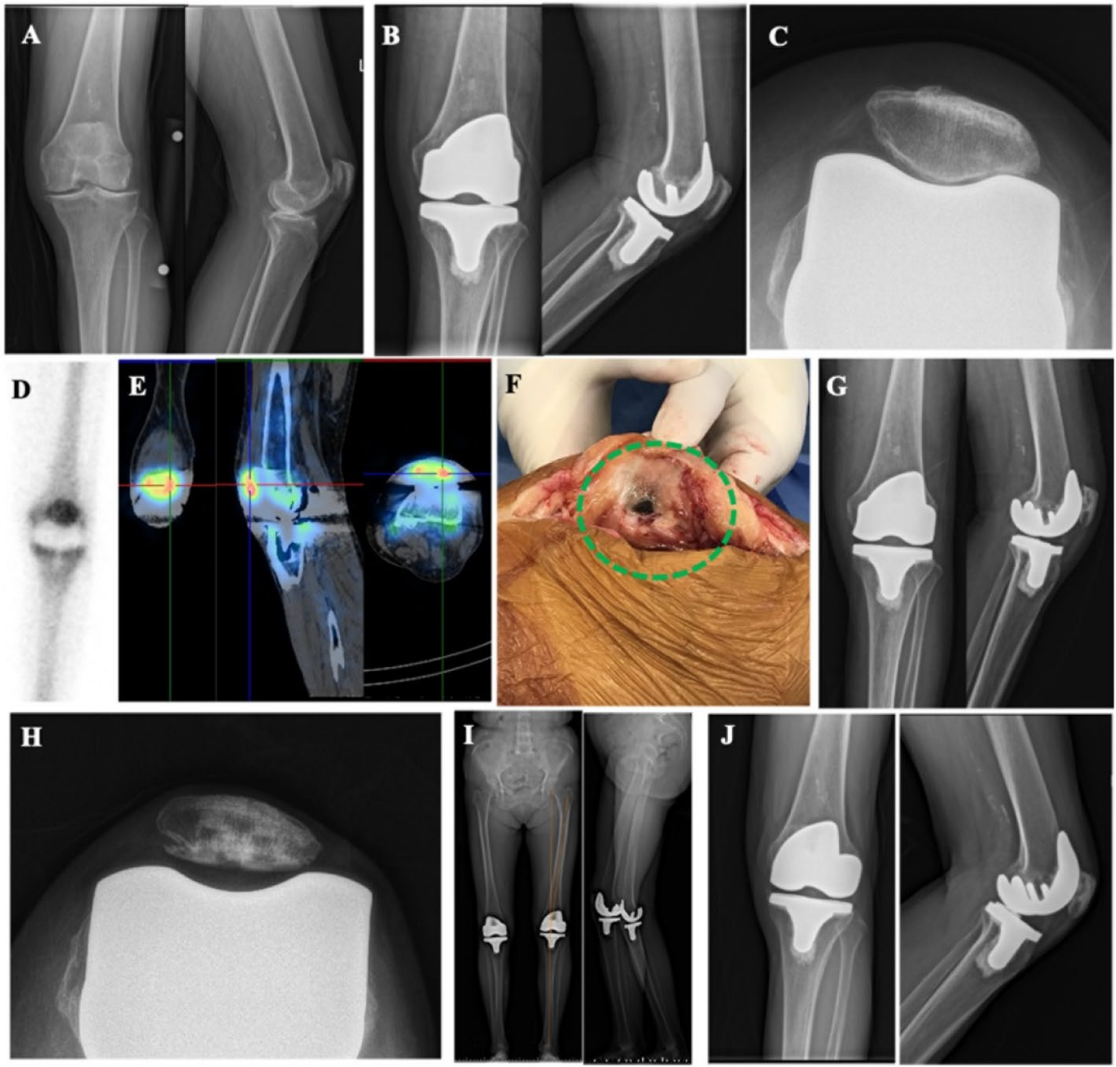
A case of SPR. A Standing anteroposterior and lateral radiographs of the left knee reveal narrowing of the medial compartment, characteristic of OA changes. B Post-TKA anteroposterior and lateral radiographs of the left knee demonstrate a well-positioned prosthesis with normal alignment. C Post-TKA axial radiograph confirms the prosthetic alignment. D Triple-phase bone scan highlights radioactive concentration within the left knee joint. E SPECT/CT imaging identifies focal radionuclide concentration on the lateral articular surface of the patella. F Intraoperative findings include impingement between the lateral articular surface of the left patella and the lateral condyle of the femoral prosthesis, along with cartilage defects and metal debris attachment. G Standing anteroposterior and lateral radiographs following SPR. H Axial radiograph post-SPR. I Full-length radiograph in functional position after SPR. J standing anteroposterior and lateral radiographs post-SPR at a two-year follow-up
